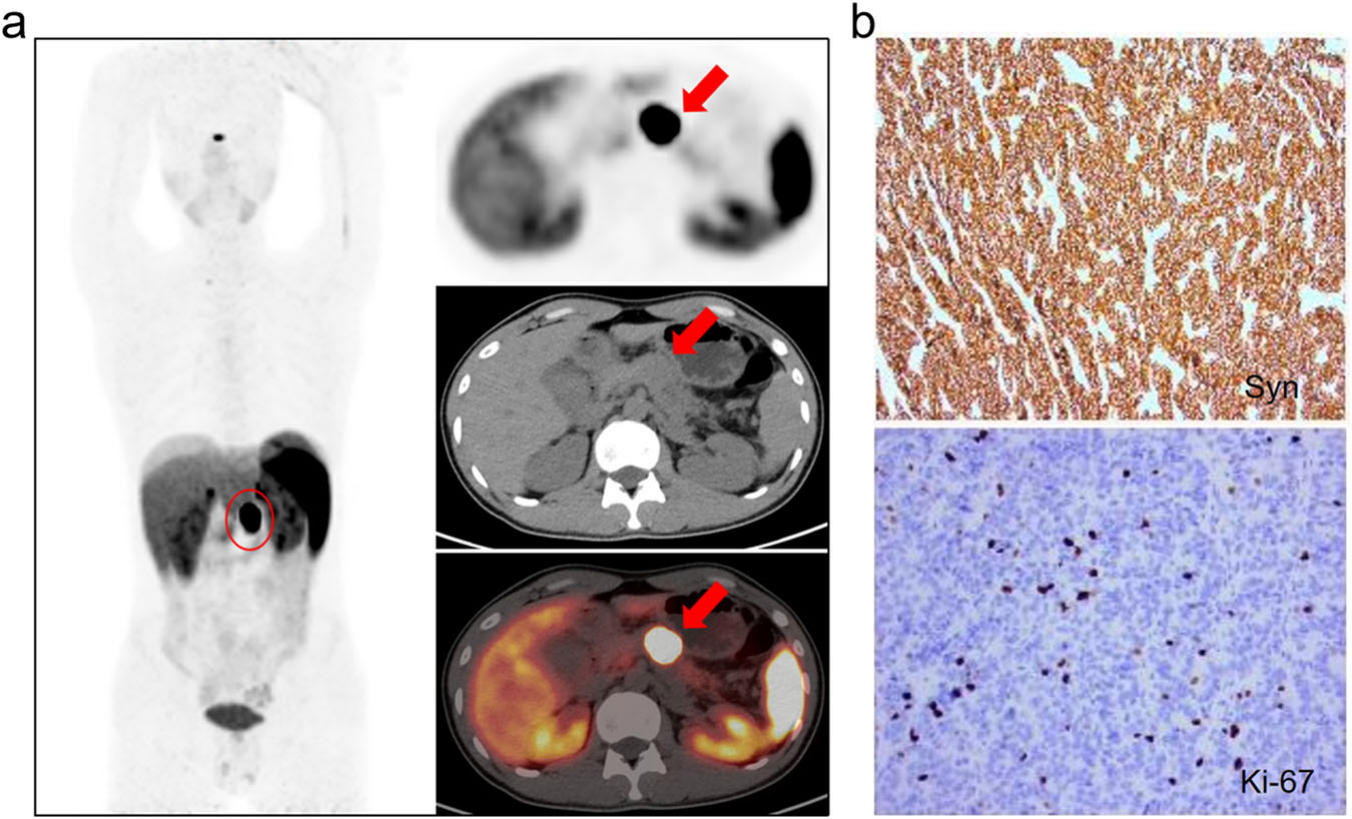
a Case report of pancreatic neuroendocrine tumor ^68^Ga-DOTATATE PET/CT revealed an isodense nodule with a high level of metabolic expression (SUVmax 56.6) in the pancreatic body. b Syn(_+_); Ki-67 (+, 10%)
